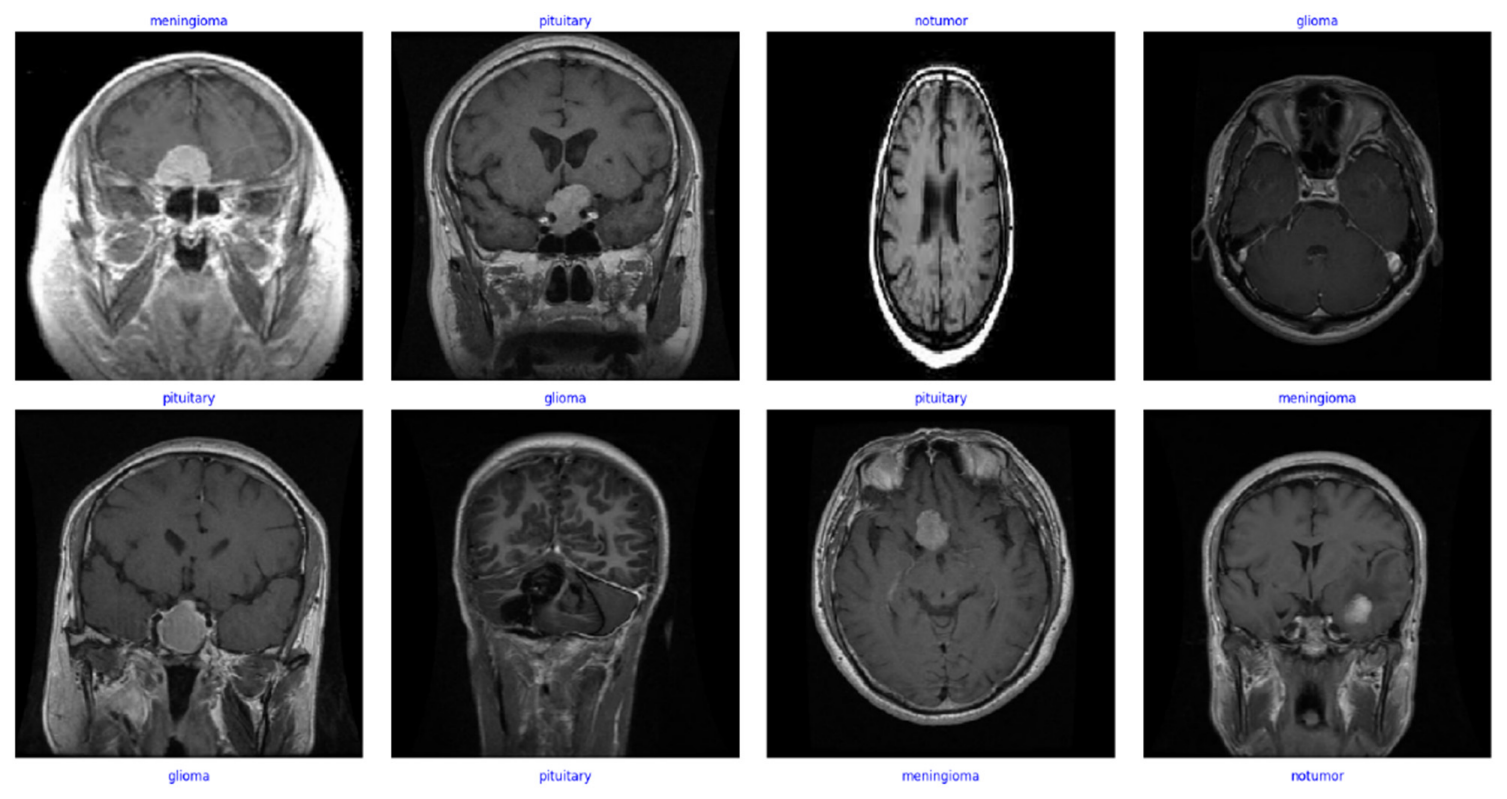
Representative preprocessed MRI samples from the heterogeneous tumor dataset.
